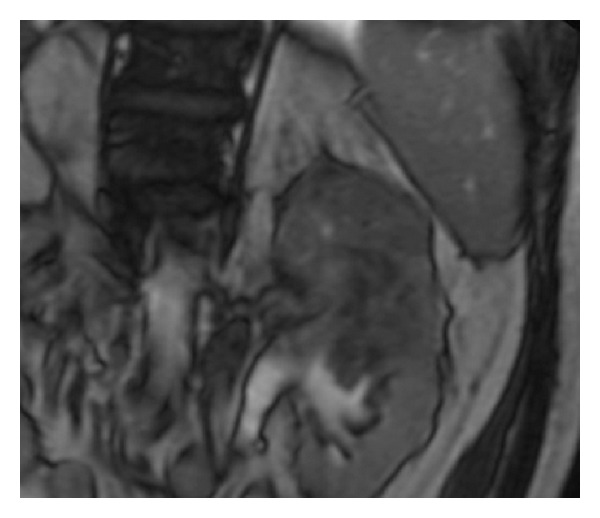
Coronal T2-weighted MRI of kidneys shows a large heterogeneous low T2 signal mass centered in the upper pole of the left kidney.
